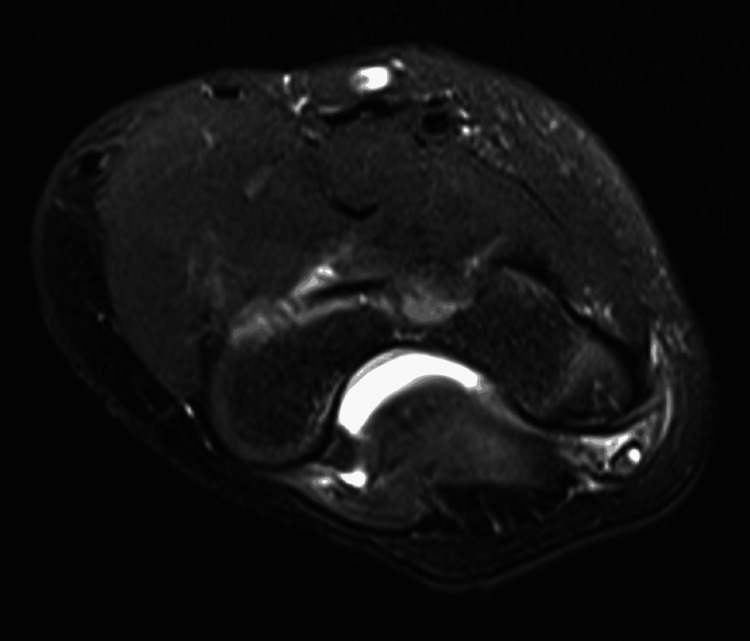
MRI of the elbow showing abnormal signal intensity with a small focal cyst likely presenting as a small tear in the ulnar nerve. MRI, magnetic resonance imaging
